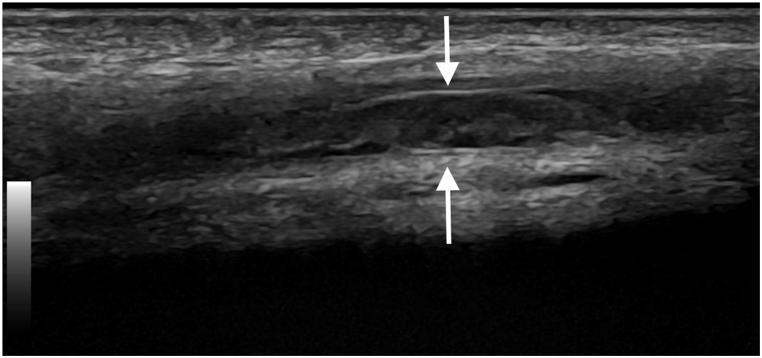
B-mode ultrasound image taken longitudinally along the dorsal vein of the penis, showing a hyperechoic thrombus distending the vein (arrows).
